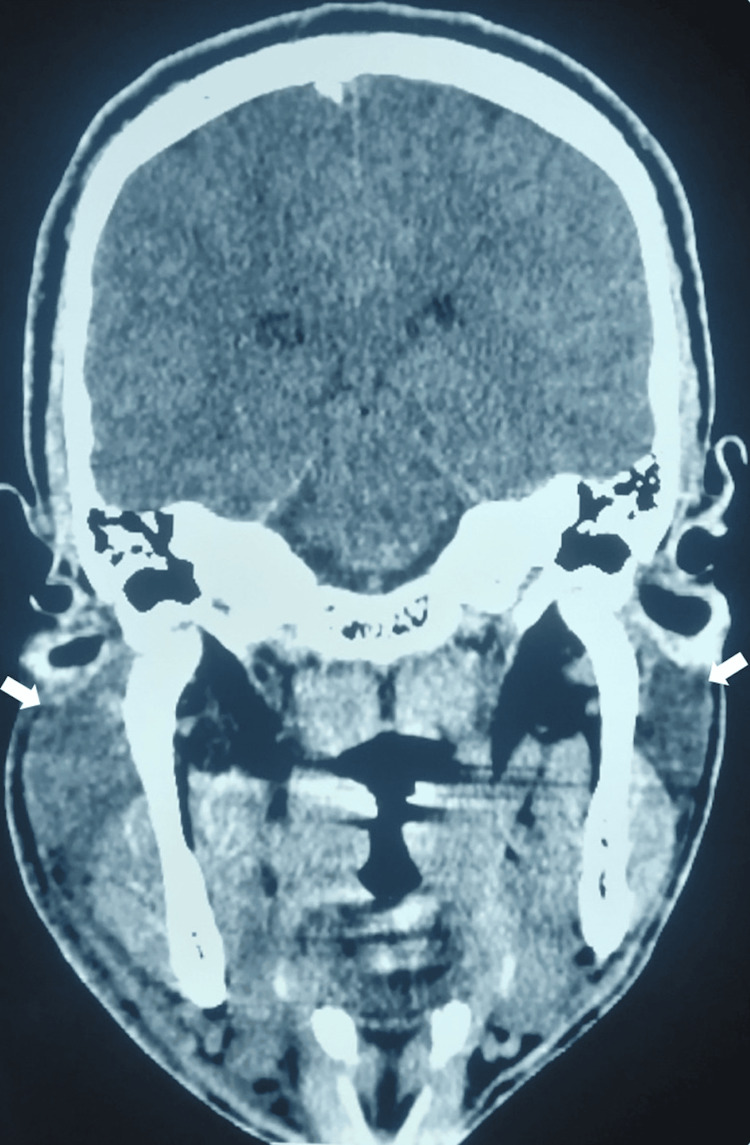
Coronal axial CT of the head demonstrating tumefaction of both parotid glands (white arrows), more prominent on the right side.
